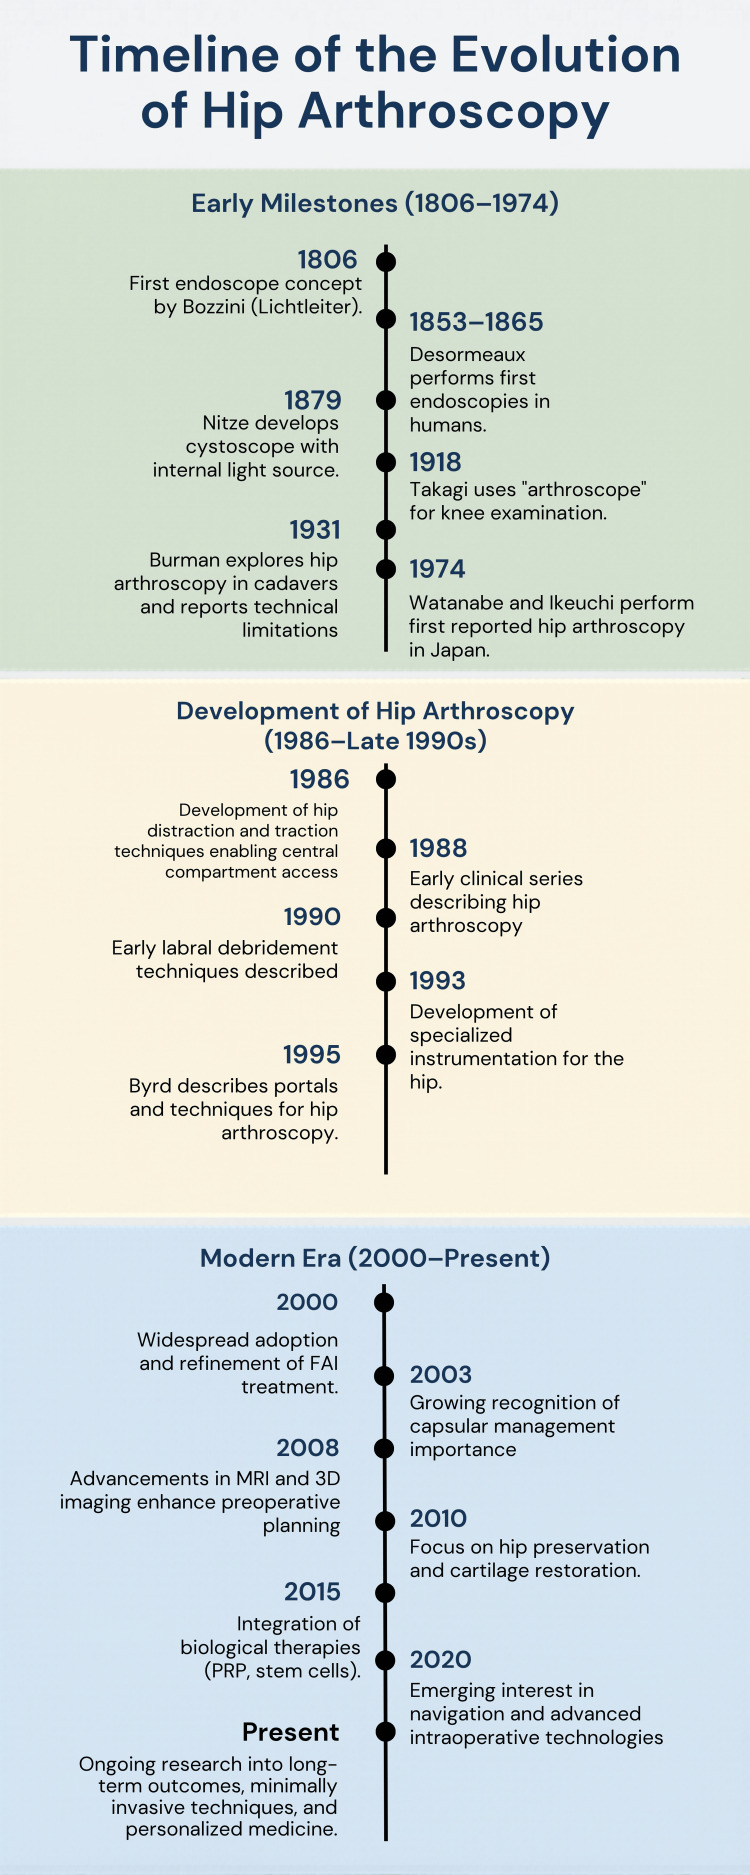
Timeline of the evolution of hip arthroscopy FAI: femoroacetabular impingement; MRI: magnetic resonance imaging; PRP: platelet-rich plasma. Figure created by Nadiya A. Persaud using Canva Pro (Canva Pty Ltd., Sydney, Australia), based on information synthesized from [2,12-30].
